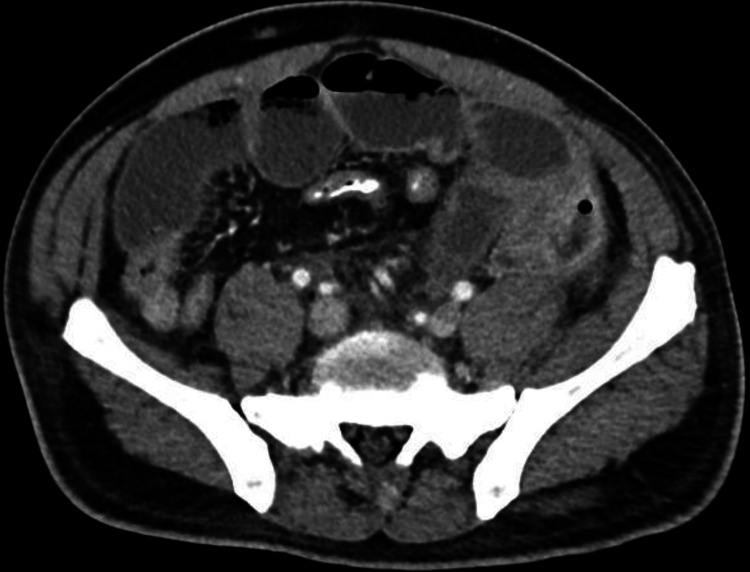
Axial contrast-enhanced CT image demonstrating an abnormal communication between adjacent small-bowel loops in the left paracolic gutter with associated mesenteric inflammatory changes and trace extraluminal fluid, findings concerning for an enteric fistula.
